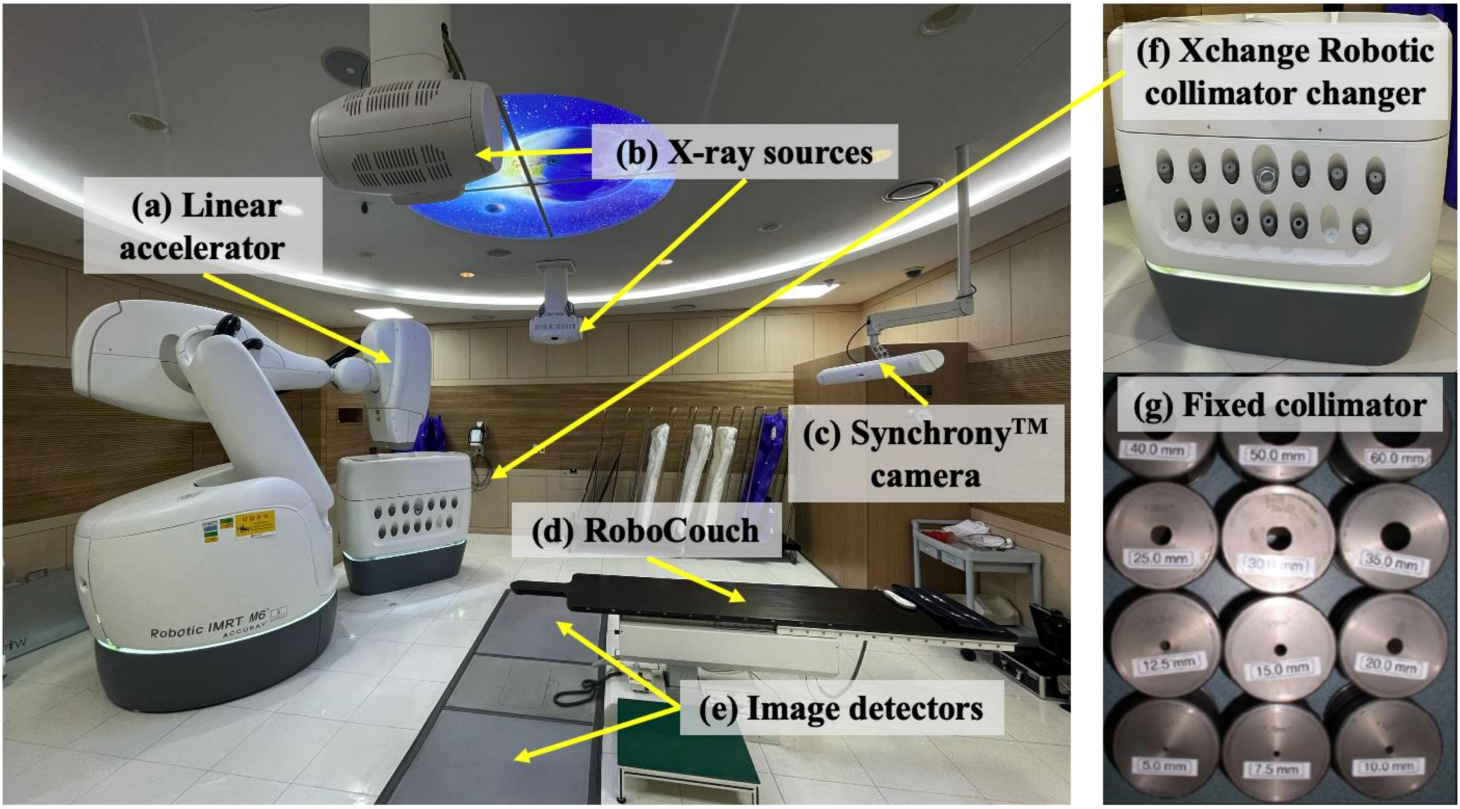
Overview of CyberKnife^®^ M6^™^ model from Accuray at Yonsei Cancer Center; (a) Linear accelerator mounted robotic arm, (b) X-ray source, (c) Synchrony™ camera, (d) RoboCouch, (e) Image detectors from x-ray source, (f) Xchanger Robotic collimator changer, and (g) different fixed collimator sizes from 5.0 mm to 60 mm.
